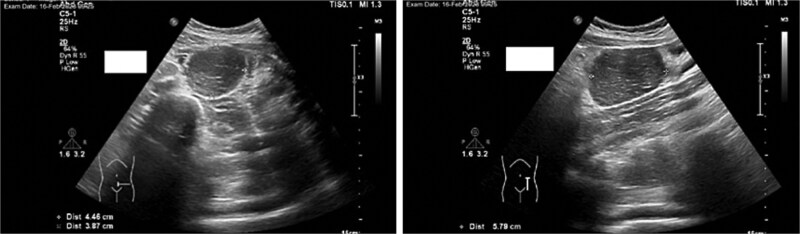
An abdominal ultrasound, which showed a hypoechoic mass in the left lower abdomen*,* and minimal free fluid.
